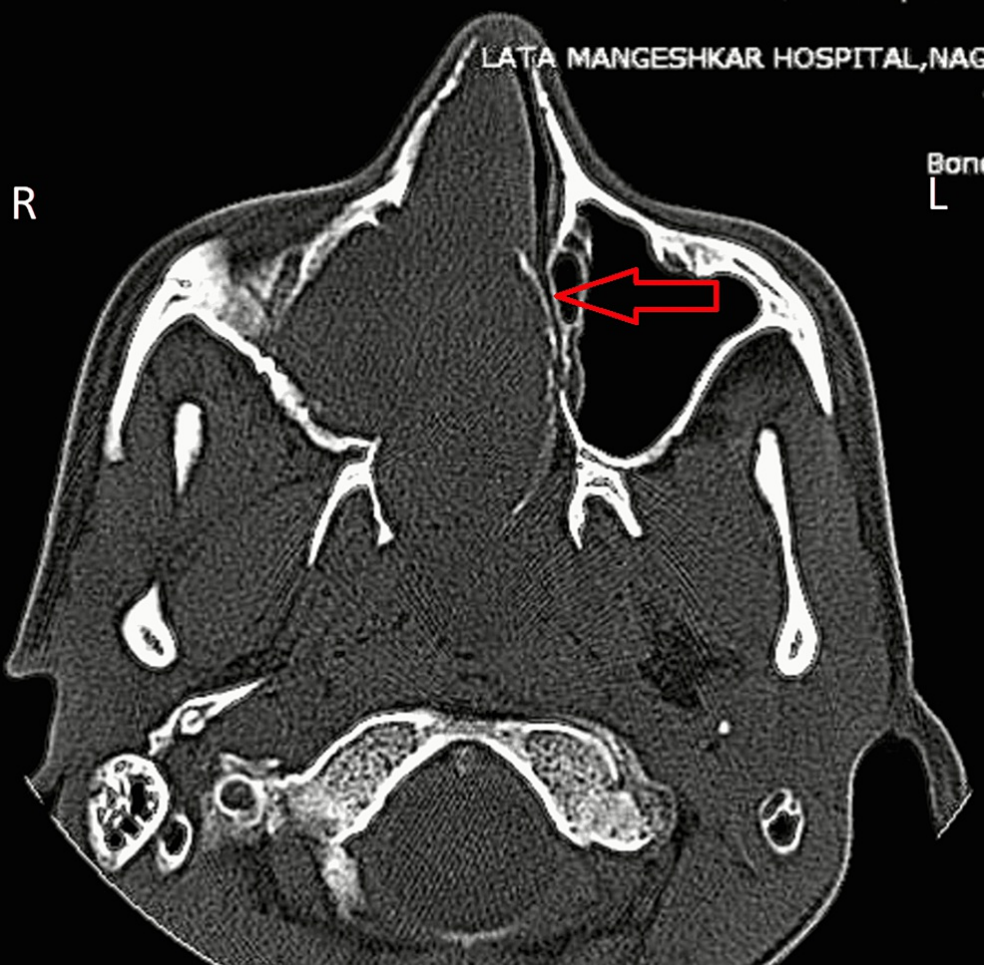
Axial plane of CT-PNS image showing, soft tissue density lesion completely occluding right maxillary sinus, extending anteriorly into the right nasal cavity through the widened ostium with mass effect on the nasal septum resulting in deviated nasal septum with convexity toward the left side and atrophy of the corresponding middle turbinate due to compression. The absence of medial wall of the right maxillary sinus with thinning of the medial pterygoid plate on the right side suggestive of bony involvement. PNS - paranasal sinuses
